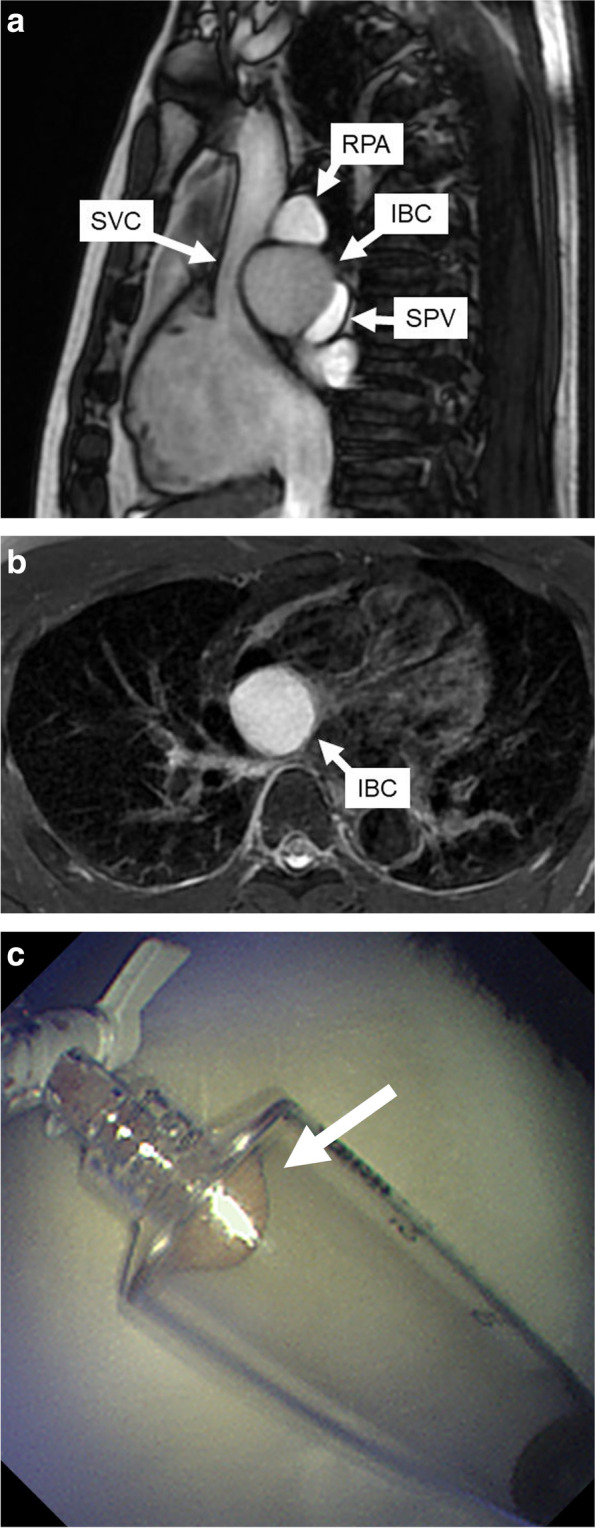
Sagittal and axial view of chest MRI, and sampling fluid by EBUS-TBNA. a The iso-intensity of the cystic mass on T1-weighted images compressing the SVC and SPV. b The high intensity of the cystic mass on T2-weighted images. c The yellowish brown mucinous fluid (white arrow)
